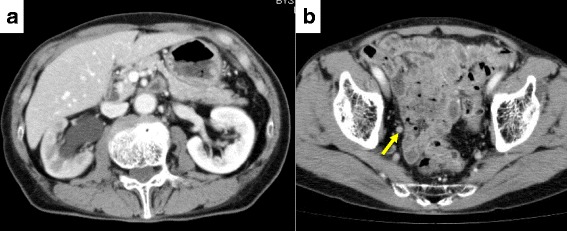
Computed tomographic findings of (a) hydronephrosis and (b) the patient’s tumor (*arrow*)
